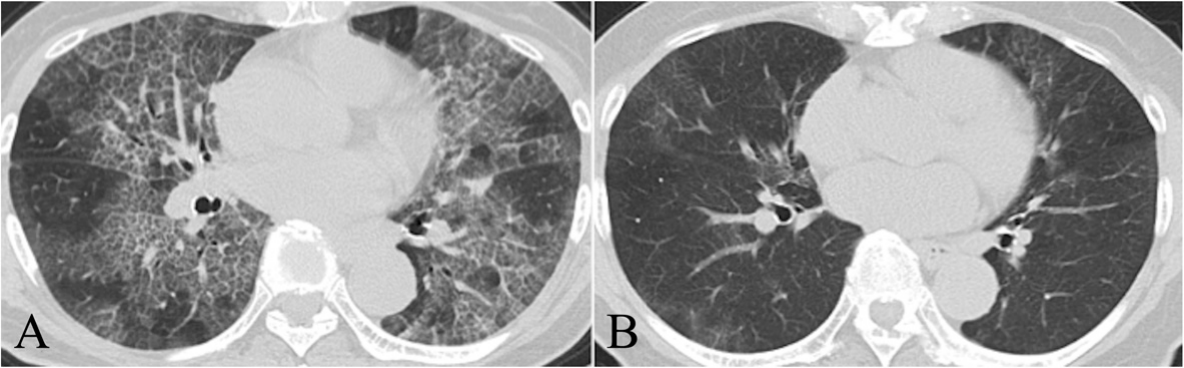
Chest computed tomography (CT) scan before and after the treatment. (A) Chest CT scan on admission. (B) Chest CT scan after five months of treatment with ambroxol showed almost no abnormal shadows.
